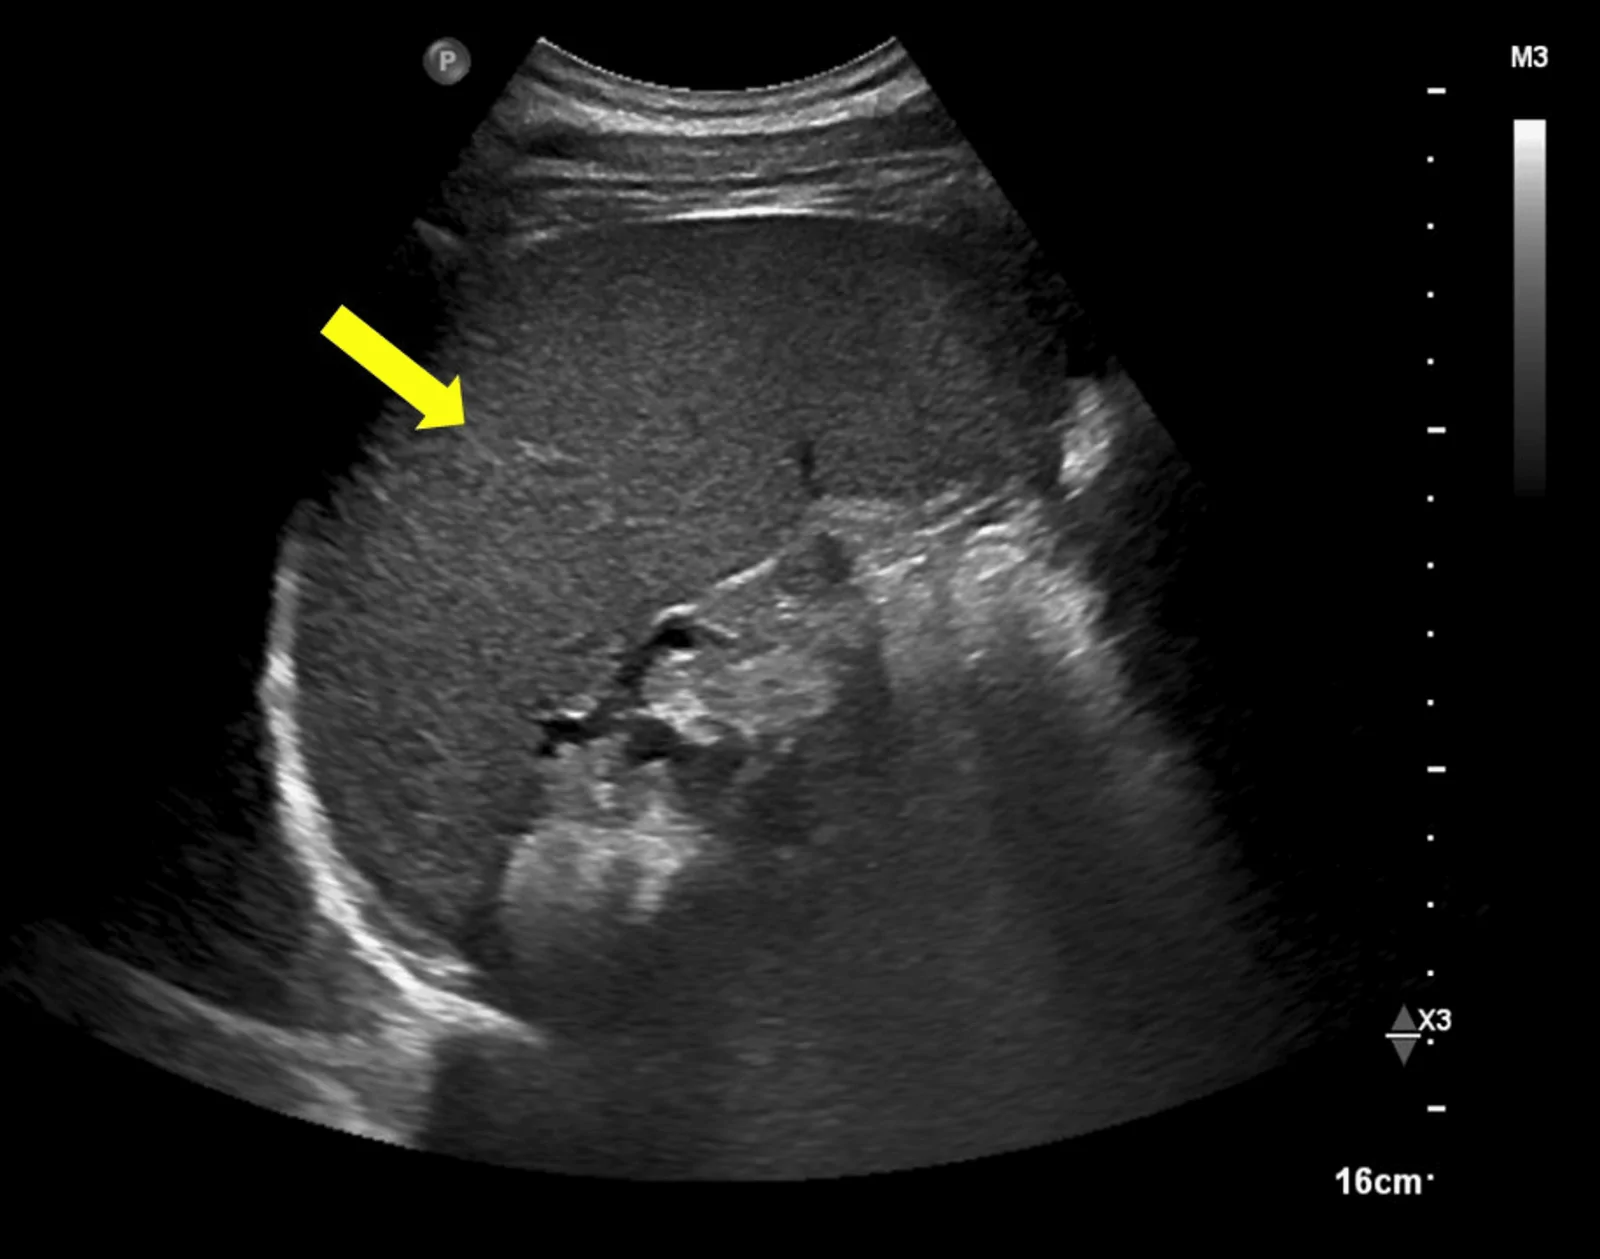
Longitudinal abdominal ultrasound demonstrating mild splenomegaly. Arrow indicates the spleen.
